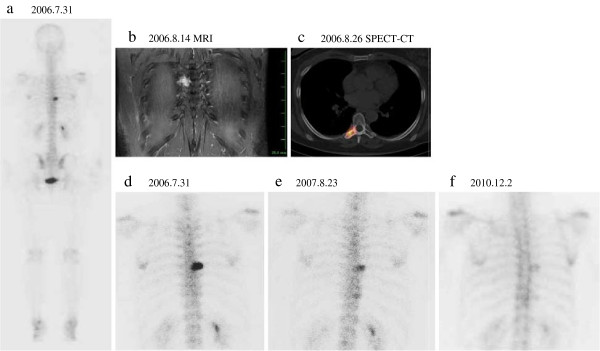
Images before and after autologous formalin-fixed tumor vaccine (AFTV) treatment, palliative radiotherapy, and adjuvant chemotherapy. a) ^99m^Tc-whole body scan, b) magnetic resonance image (MRI), and c) single photon emission computed tomography (SPECT-CT) image before surgical resection of the primary tumor on 31 June 2006. d) Expanded image of ‘a’ at the site of vertebra Th7 on 31 June 2006, e) 1 year later, and f) 4 years later at the same site.
